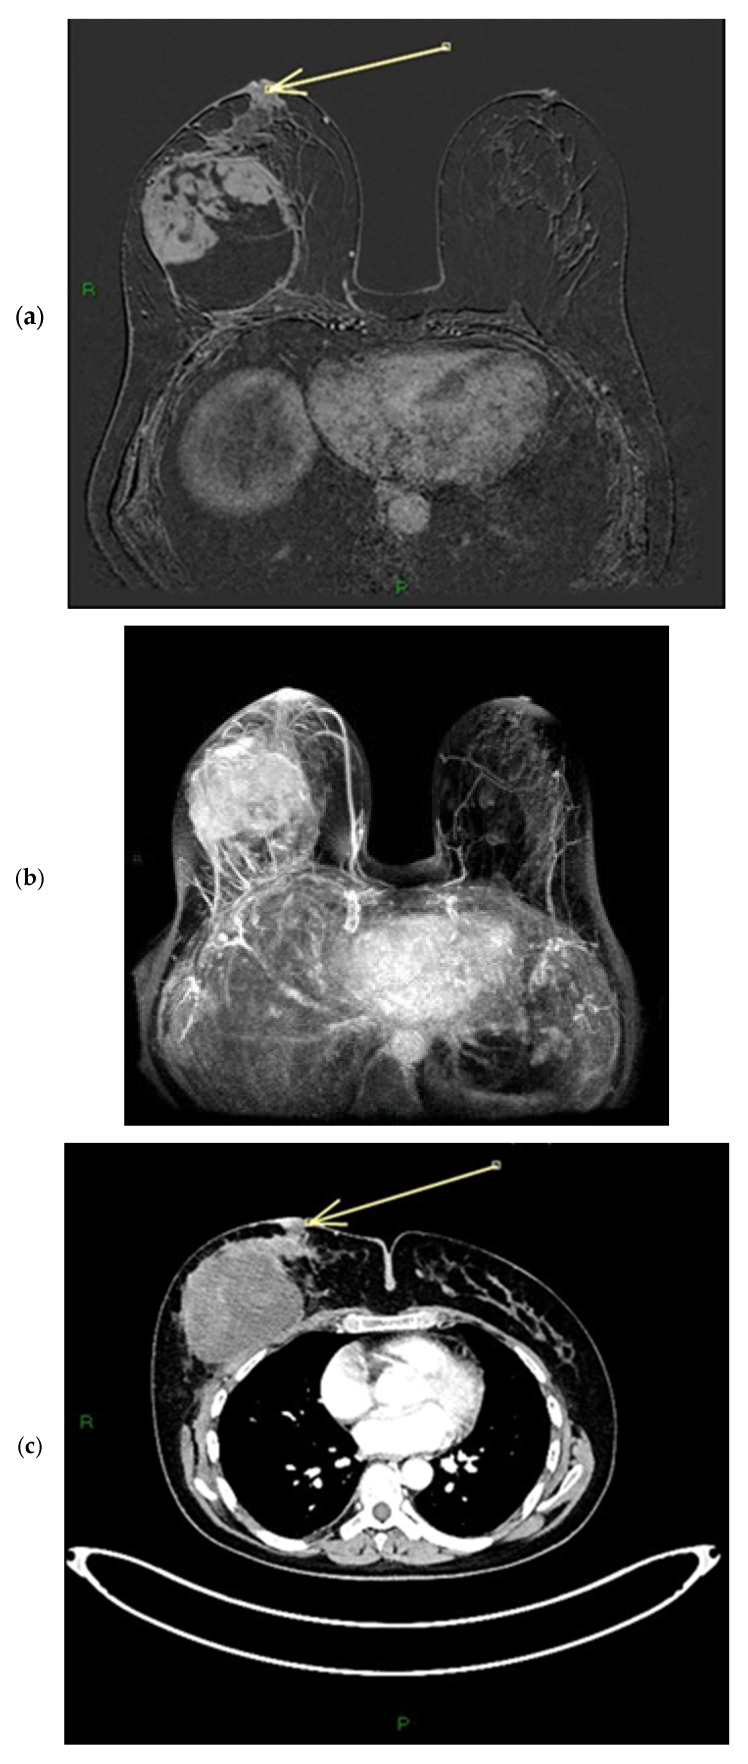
(a–c) Patient’s name V.F. During ce-MR, we notice a flat thickened right nipple, with the representation of SLE, NEZ, and marked patchy INE pattern of enhancement (a). In the ce-T1 sequence (a) and in the MIP reconstruction (b), we can observe the presence of a partly necrotic mass-like enhancement of at least 6 cm located in the external quadrants of the right breast; the non-mass-like enhancement component that infiltrates the nipple measures approximately 3 cm and extends anteriorly to the gross mass (arrow in a). In the ce-CT scan, the arrow shows enhancement of both the base and the body of the right nipple. Morphology is flat, with skin thickening associated (c).
